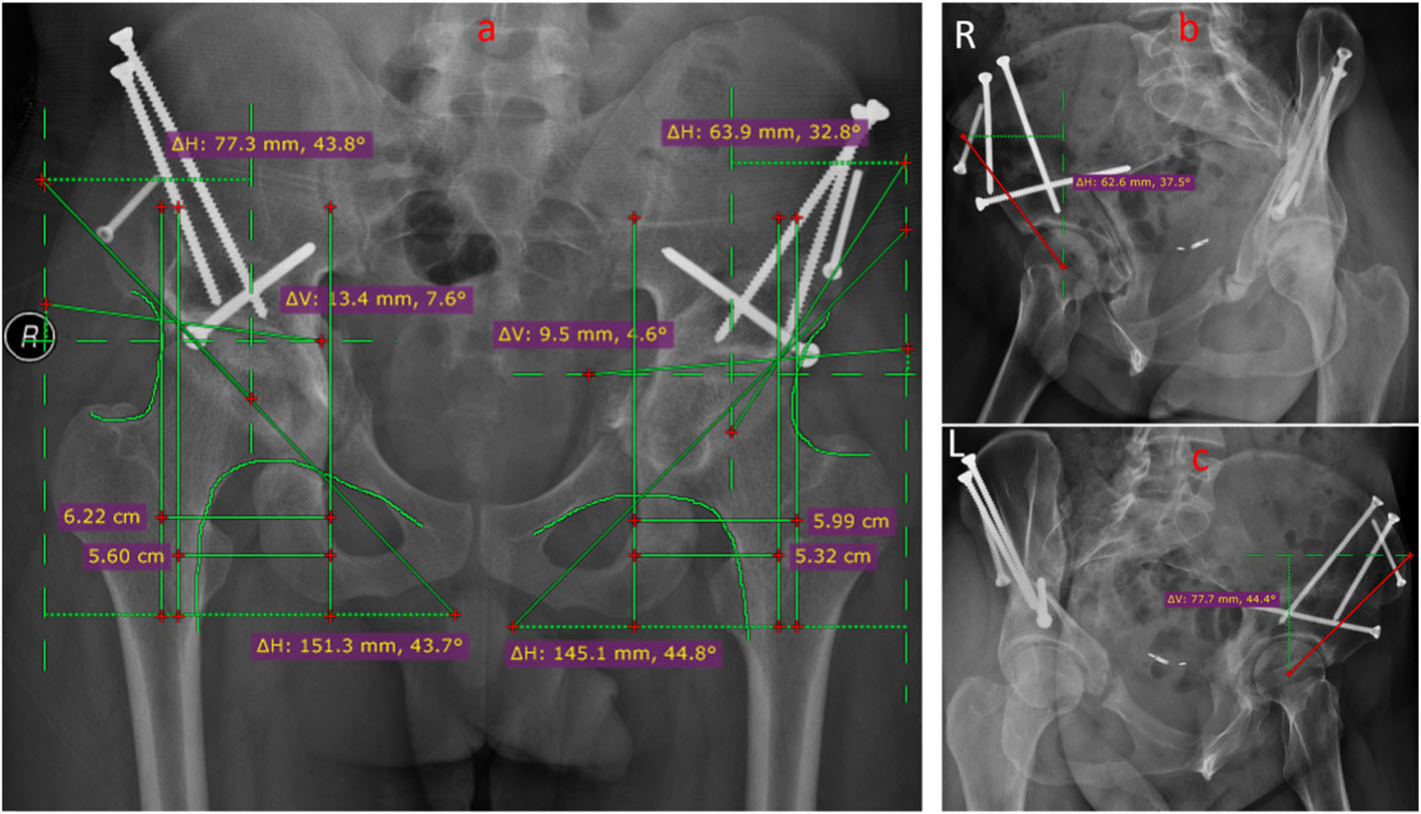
Radiographic images of the hip at post-operation. a Pelvic positive position. b Left hip joint 65° oblique piece at post-operation. c Right hip joint 65° oblique piece at post-operation
